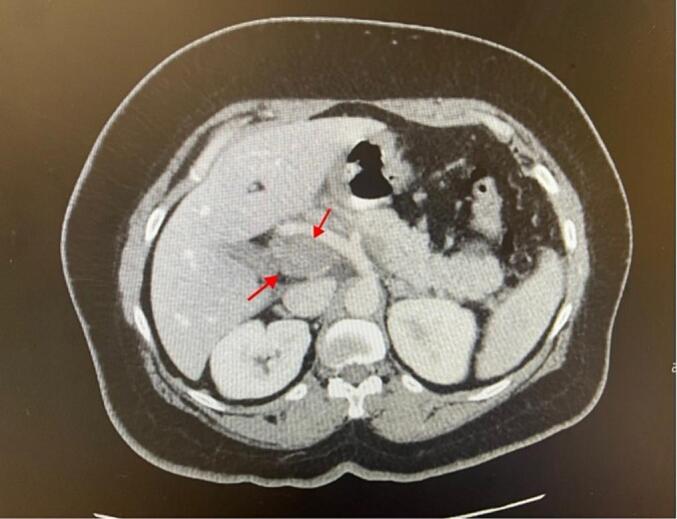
CT Abdomen and Pelvis with Contrast showed the extensive portal vein thrombosis (red arrows). (For interpretation of the references to colour in this figure legend, the reader is referred to the web version of this article.)
